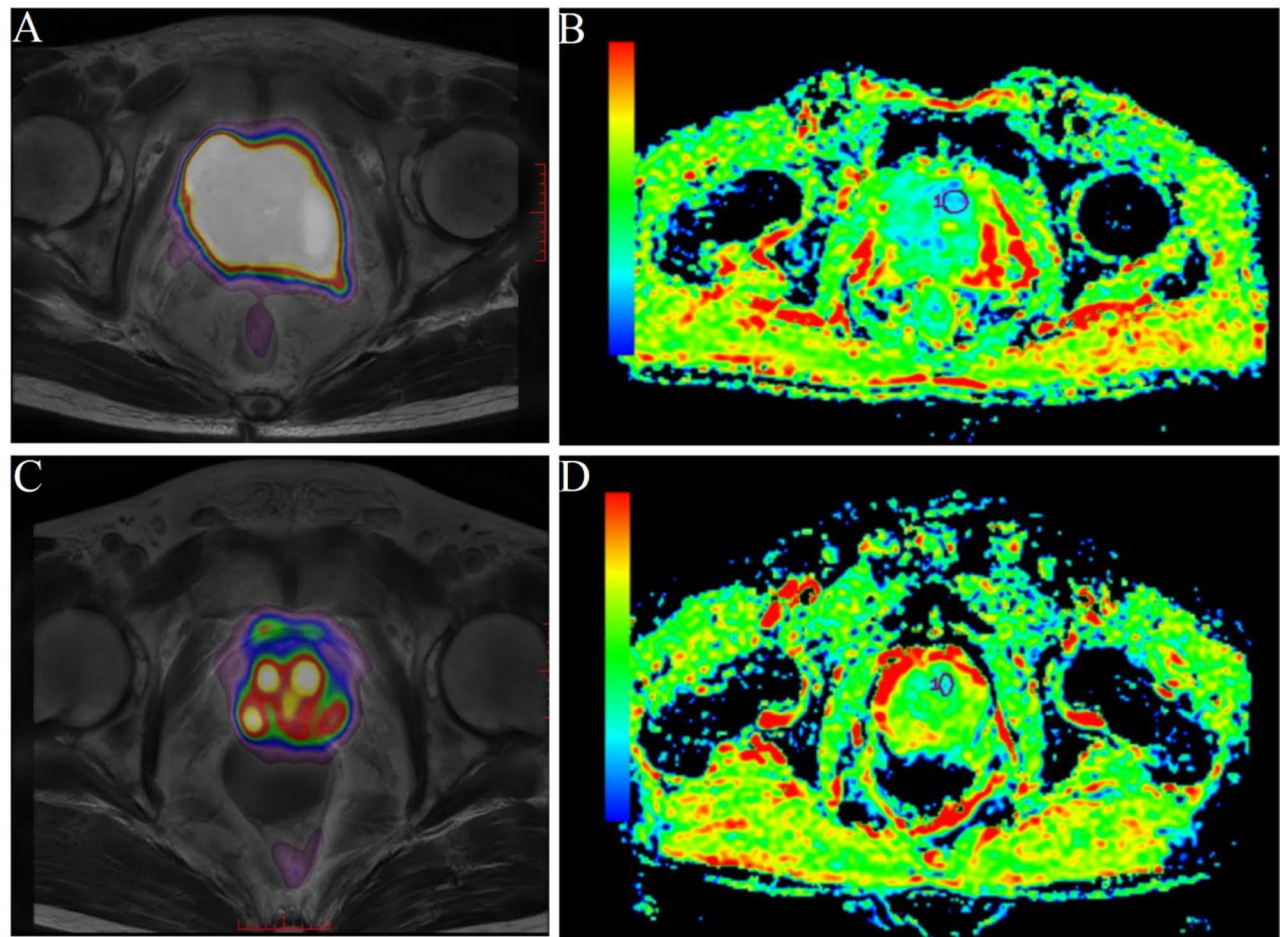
A high-risk prostate cancer patient had positive Ki-67 with 30%. ^68^Ga-PSMA-11 PET/MR showed the lesion had intense focal ^68^Ga-PSMA-11 uptake with an SUV_max_ of 18.34 (A) and corresponding standard ADC value was 0.000731(B). However, a low-risk patient with Ki-67 of 2%, whose SUV_max_ and standard ADC was 7.49 and 0.00135, respectively (C and D).
